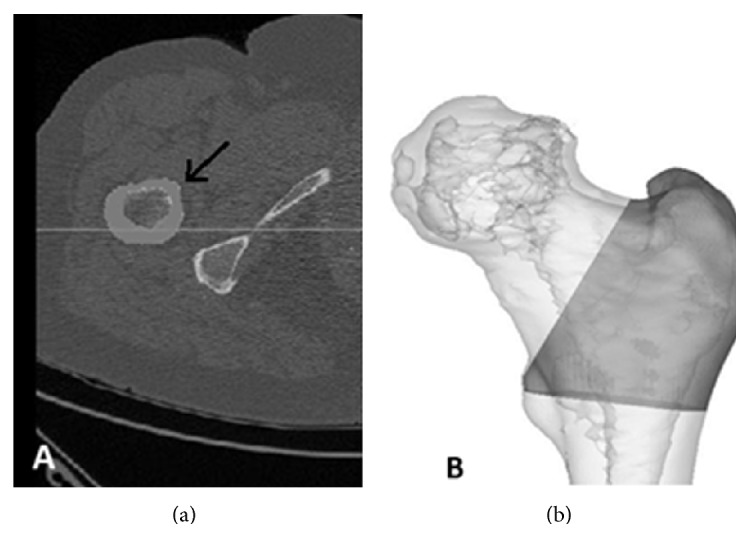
(a) Axial view of one slice of the CT-data from a patient. The arrow points to the area belonging to the mask. (b) The 3D view of the region of interest for BMD calculations.
